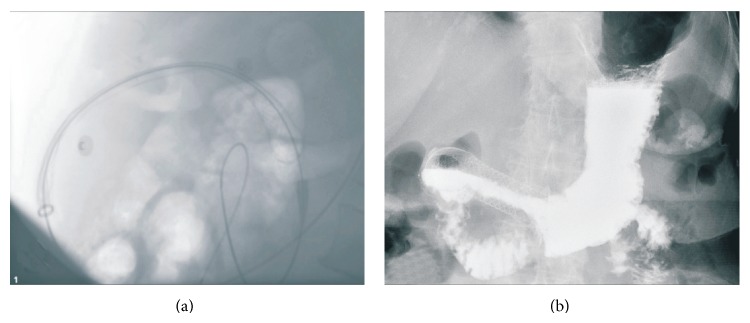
X-ray images of stents. (a) Image showing a stent released. (b) An example of barium contrast radiography of a funnel stent.
